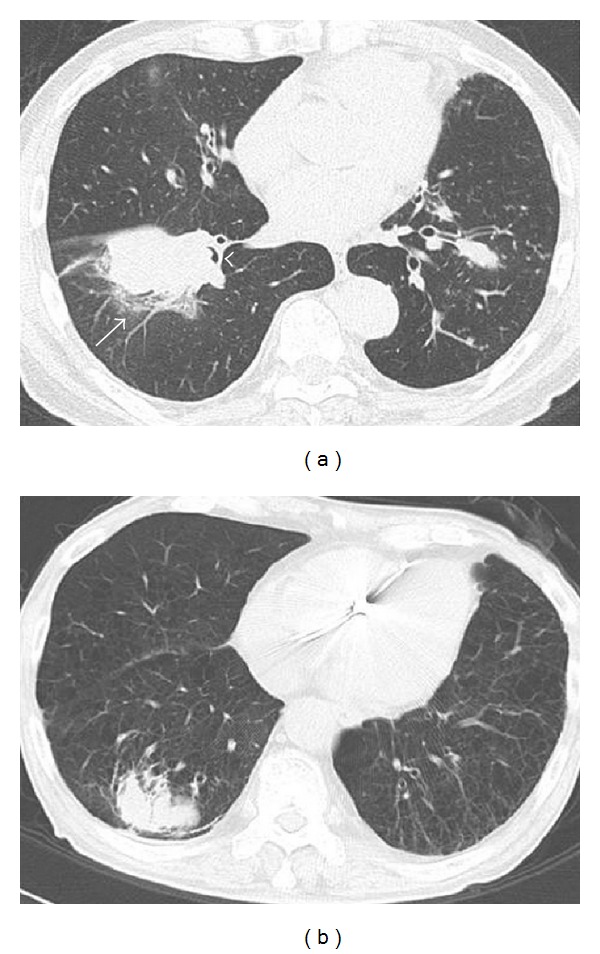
CT findings of the central and peripheral squamous cell carcinomas. (a) Central squamous cell carcinoma was defined as a lesion located from trachea to the segmental bronchi. Note bronchiolar obstruction (arrowhead) and peripheral obstructive pneumonia (arrow). (b) Peripheral squamous cell carcinoma was defined as the one located in the more peripheral location.
